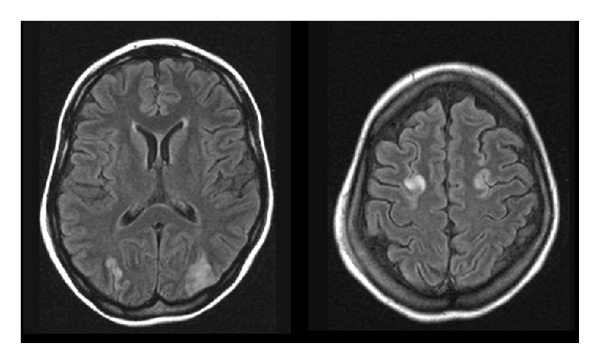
Brain MRI-FLAIR showing an increase of signal in both occipital and frontal lobes.
